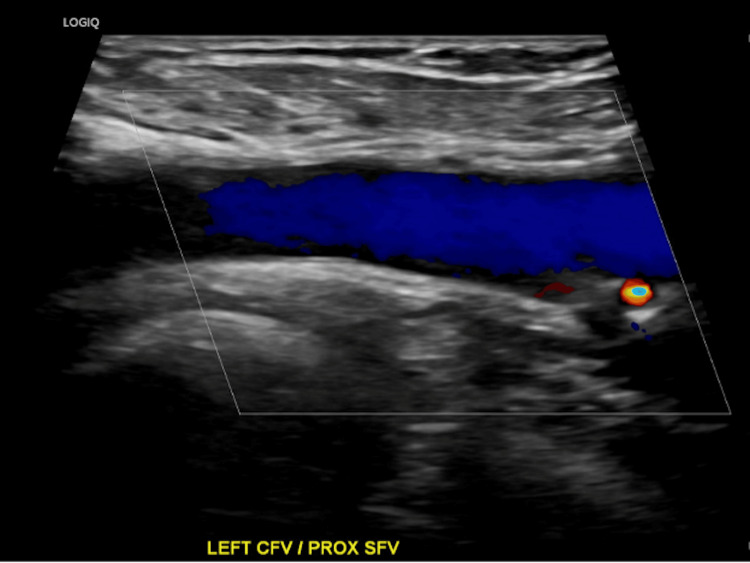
Doppler ultrasound image of the left common femoral vein (CFV) and proximal superficial femoral vein (SFV) demonstrating normal venous blood flow in the left lower limb.
